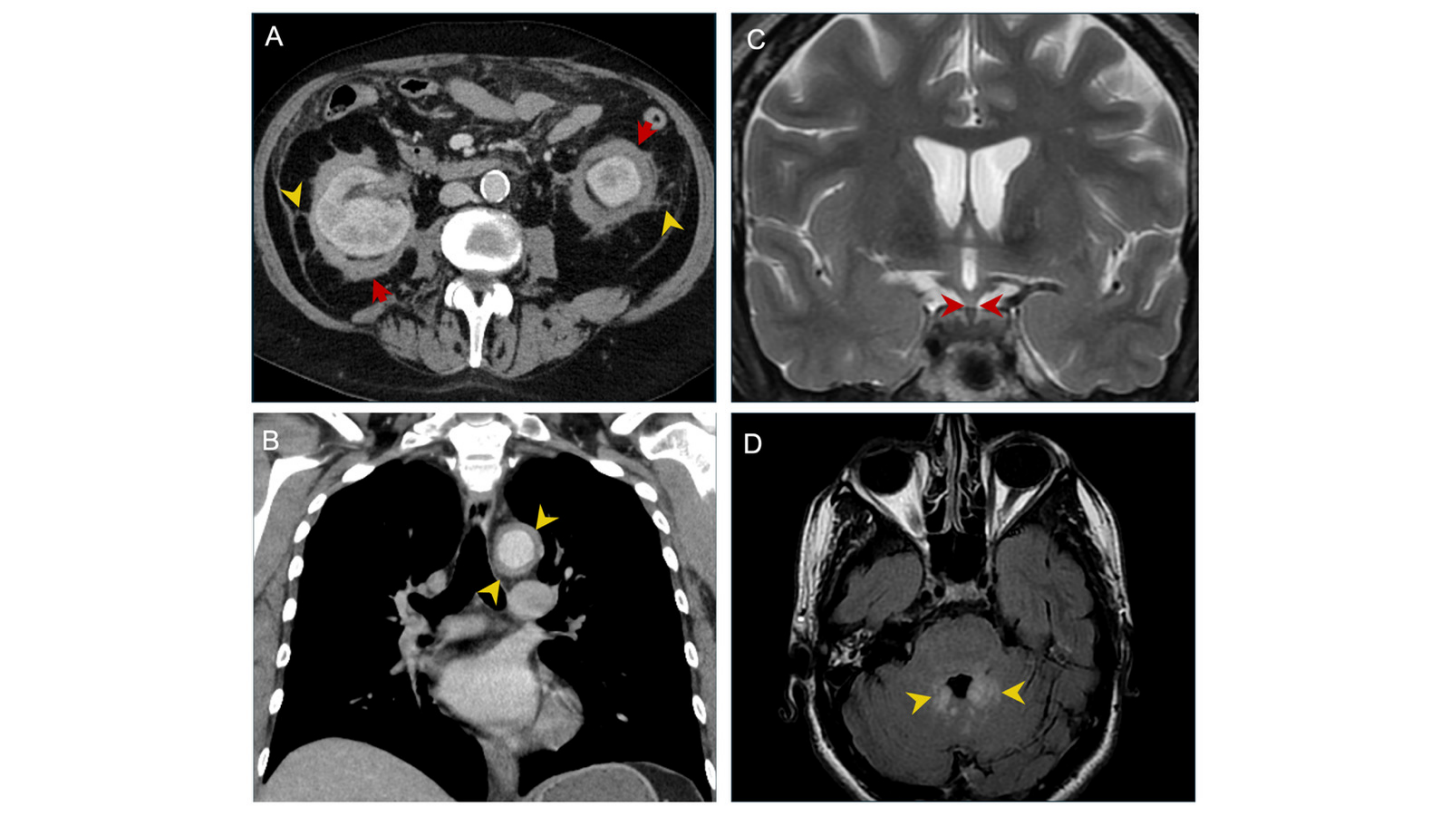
The retroperitoneal (A), cardiovascular (B), and neurologic (C, D) manifestations of ECD. A: Axial portal venous phase CT demonstrating soft tissue infiltration (yellow arrows) and perirenal septal thickening (arrowheads) known as the pathognomonic ‘hairy kidney sign’ [Case 4]. B: Coronal contrast-enhanced CT demonstrating the characteristic circumferential infiltration of the aorta (arrowheads) known as the ‘coated aorta sign’ [Case 3]. C: Coronal T2-weighted MRI demonstrating a thickened pituitary infundibulum (arrowheads) [Case 5]. D: Axial T2 FLAIR hyperintensities at the bilateral dentate nuclei within the cerebellum (arrowheads) [Case 5].
